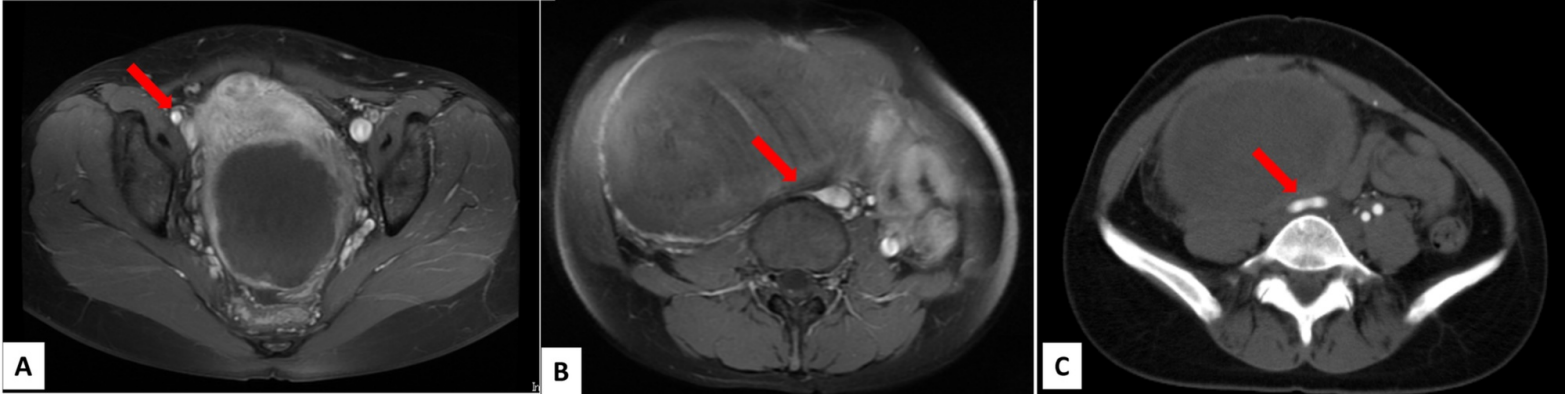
Contrast-enhanced T1-weighted images and contrast-enhanced CT images one month later. (A) Contrast-enhanced T1-weighted images show enhancement of right external iliac artery (arrow). (B) Right common iliac artery is flattened and unclear (arrow). (C) Contrast-enhanced CT images show patency of right common iliac artery.
